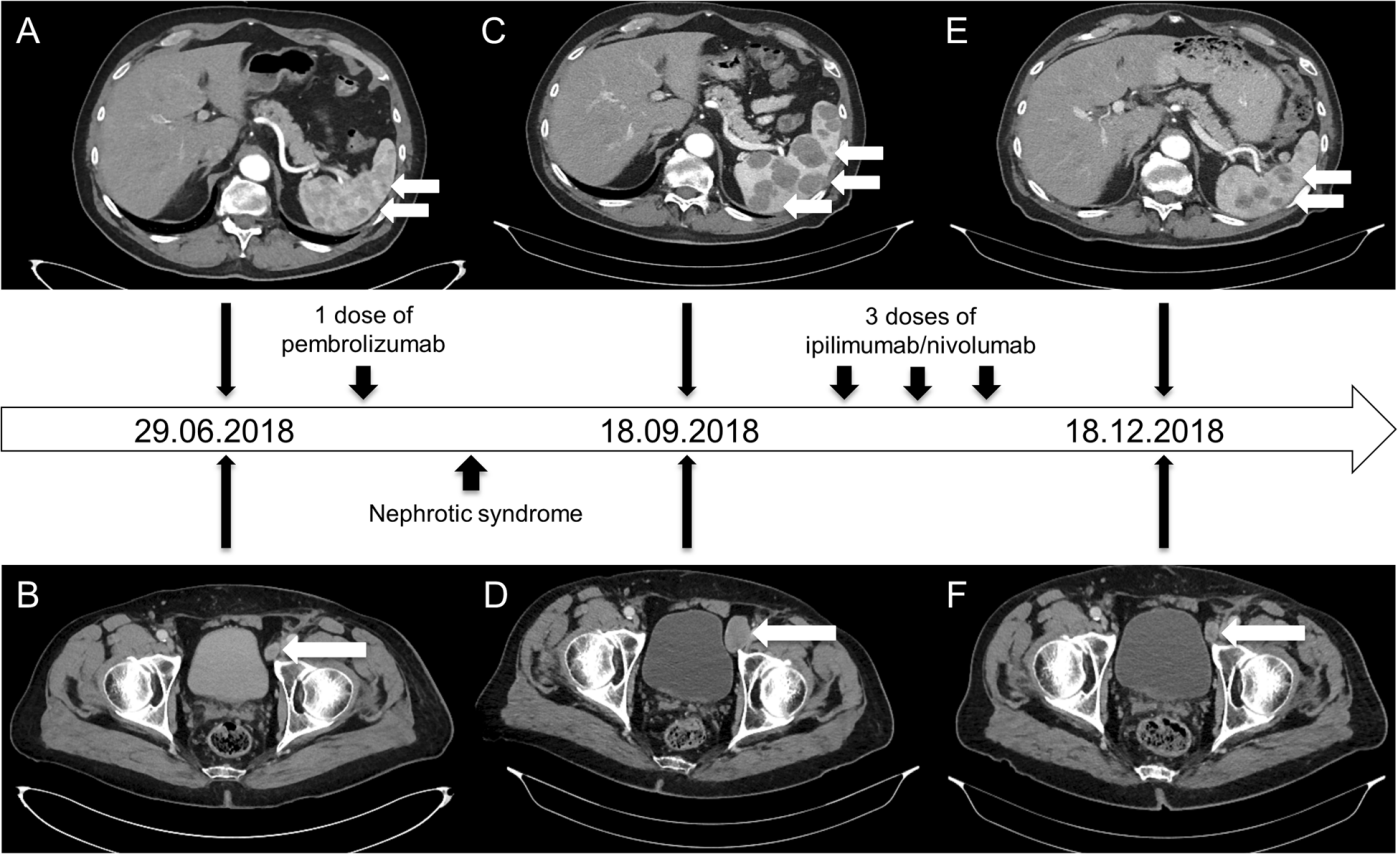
Timeline: a-b CT scans of the abdomen with splenic metastases and a iliac lymph node metastasis before the first dose of pembrolizumab. c-d CT scans of the abdomen with splenic metastases and a iliac lymph node metastasis after one dose of pembrolizumab and acute kidney injury. e-f CT scans of the abdomen with a fulminant response of the splenic metastases and the iliac lymph node metastasis after three doses of ipilimumab/nivolumab. White arrows indicate metastases
